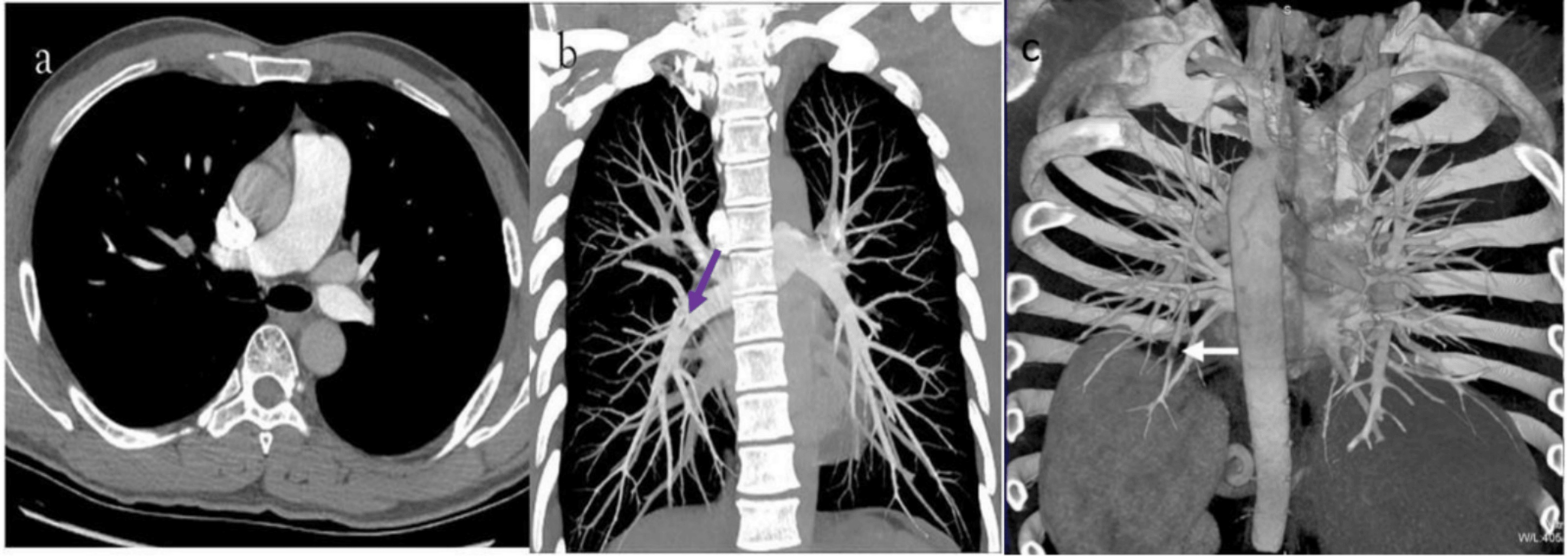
(a-c) Case 1 CTPA post-processing images CTPA (a) axial, (b) coronal MIP reconstruction (violet arrow), and (c) coronal VR reconstruction (white arrow) showing the presence of posterior filling defect of pulmonary segmental and subsegmental arteries CTPA: computed tomography pulmonary angiography; MIP: maximum intensity projection; VR: volume rendering
